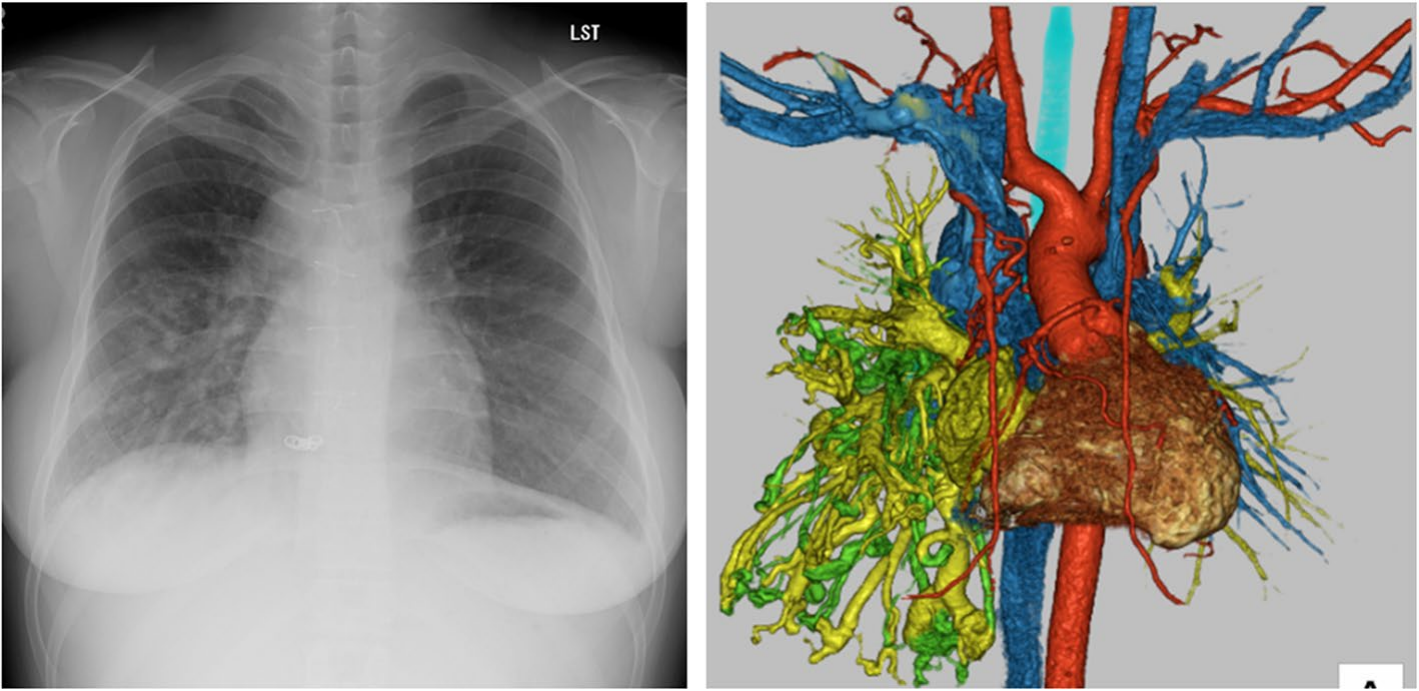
Preoperative chest images. Chest radiography taken 4 days before the operation (left) and three-dimensional computed tomography angiography (right) showing increased vascular shadows in the right lung field and presence of a pulmonary arteriovenous fistula, respectively. The red structures represent arteries, the blue structures represent veins and the left pulmonary arteries, the green structures represent the right pulmonary arteries, and the yellow structures represent pulmonary veins and the left atrium
